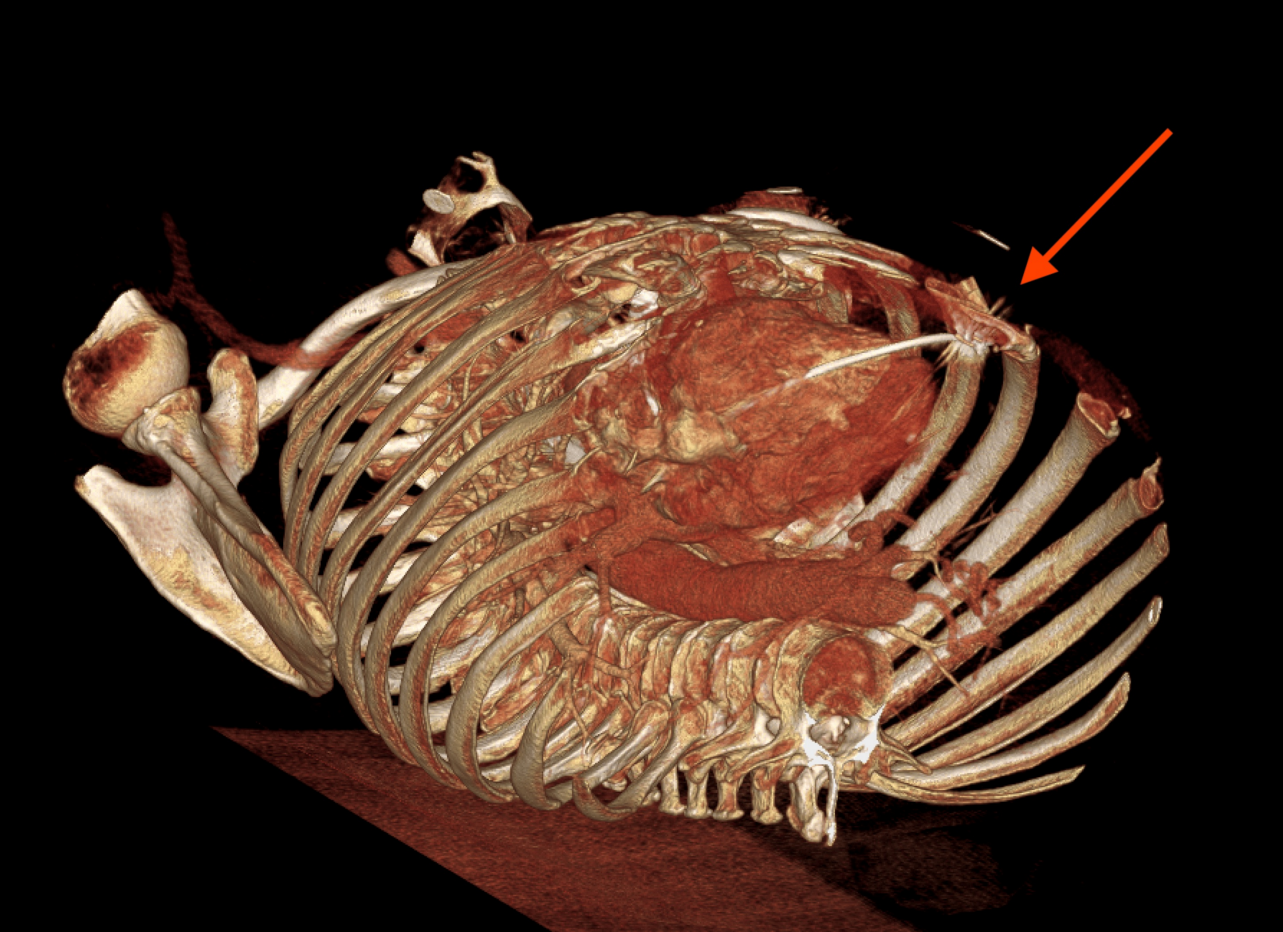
Three-Dimensional Visual of Right Ventricular (RV) Lead Perforation 3-D reconstruction from chest CT imaging, showing a caudo-cranial view of RV lead perforation. Red arrow pointing to distal lead tip within the anterior chest wall after puncturing through RV wall. Image created using Visage Imaging Software (Visage 7 platform, version 2.5.0; Visage Imaging, Inc., San Diego, CA, USA) by Dr. Jeremy Williams.
